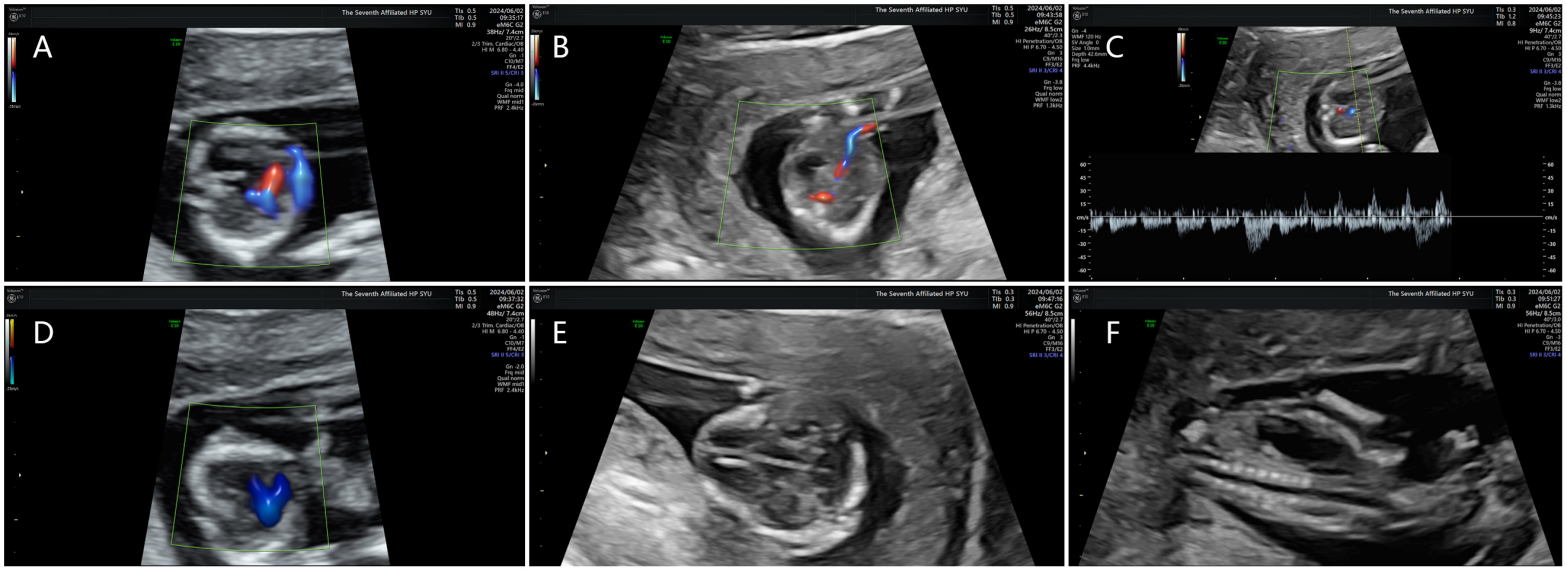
Diverse fetal anomalies identified on prenatal ultrasound. (A) Ventricular deficiency. (B) Abnormal development of venous ducts. (C) Intravenous catheterization spectrum reverse a-wave. (D) Common trunk of great arteries. (E) abnormal cerebellar development. (F) left congenital diaphragmatic hernia.
